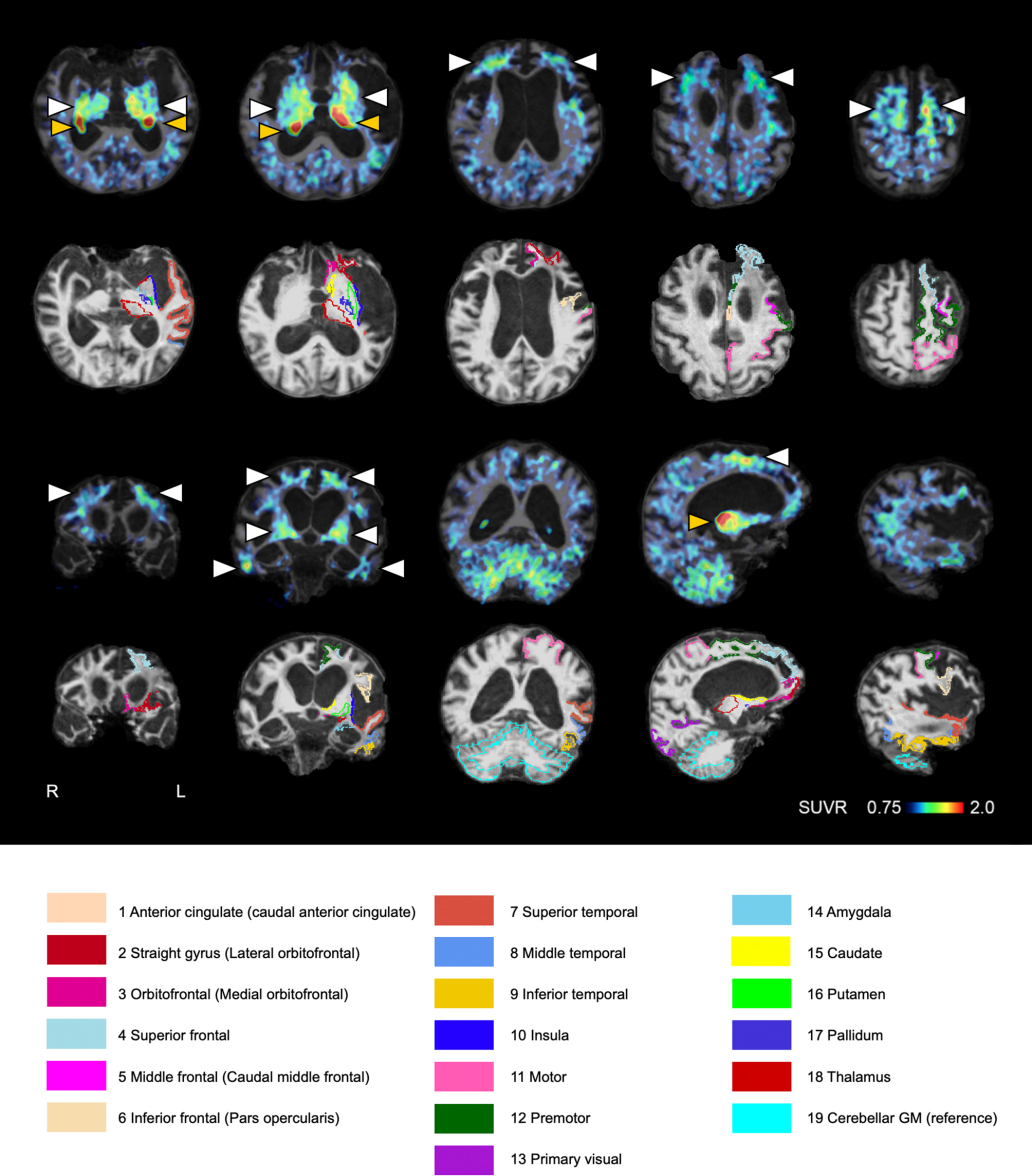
Images of ^18^F-florzolotau PET SUVR, along with T1-weighted MRI and ROIs. SUVR Images of ^18^F-florzolotau PET are shown along with T1-weighted MRI images. White arrowheads indicate enhanced parenchymal radioligand retention, and yellow arrowheads denote radioactivity accumulations in the choroid plexus supposedly unrelated to tau depositions. ROIs except for the cerebellar gray matter reference are for the left hemisphere only. Only the outermost boundary of each ROI is outlined. Abbreviations: ROI, region of interest; SUVR, standardized uptake value ratio
